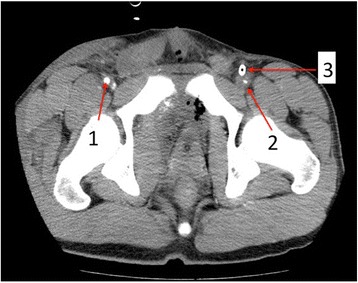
Enhanced computed tomography imaging after the primary surgery. *Arrow 1*: right femoral artery (size 5.6 mm). *Arrow 2*: left femoral artery. It was not enhanced due to sheath placement. *Arrow 3*: 12-Fr sheath placed in the left femoral artery. Lack of enhancement of the left femoral artery indicates lack of blood flow
